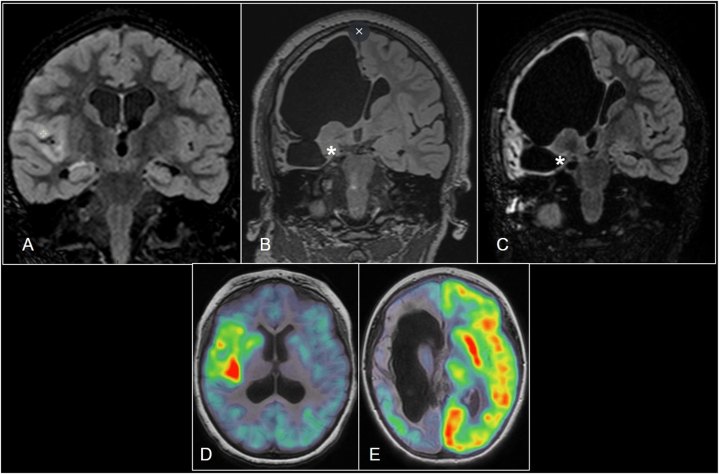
Patient 1 modified coronal sections using FLAIR sequences showing hypersignal in the peri- insular cortex on pre-hemispherotomy MRI (A); possible persisting connection exiting the right amygdala marked by a “*” on pre-amygdalectomy MRI (B); complete resection of right amygdala marked by a “*” on post-amygdalectomy MRI (C); ¹⁸F-fluorodeoxyglucose (¹⁸F-FDG) PET scans fused to T1 weighted-MRI showing pathological hyperactivity in the right peri-insular region on the pre- hemispherotomy imaging (D); resulting hypoactivity in the right peri-insular region compared to the normal activity of the left hemisphere on the pre-amygdalectomy imaging (E).
